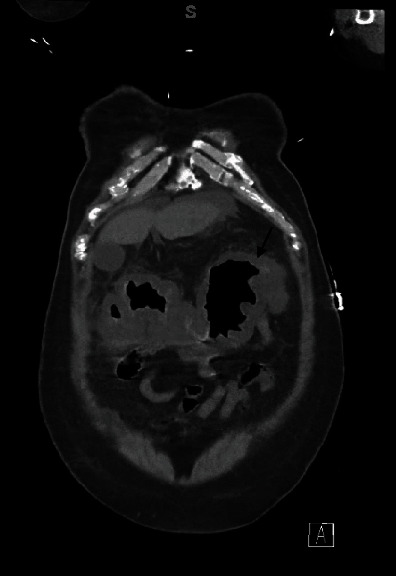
CT abdomen coronal view: arrow indicating thumbprinting sign indicative of colitis in a patient having coinfection with COVID-19 and Clostridium difficile.
